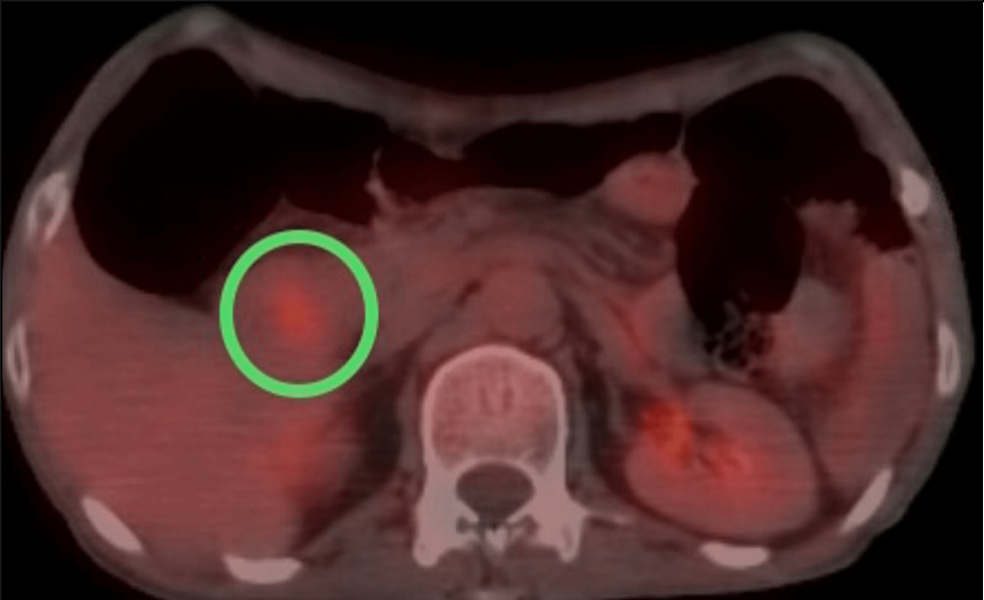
PET-CT. Cross-sectional PET-CT showing an increased FDG-avid activity in the second part of the duodenum. PET-CT = positron emission tomography-computerized tomography; FDG = fluorodeoxyglucose
